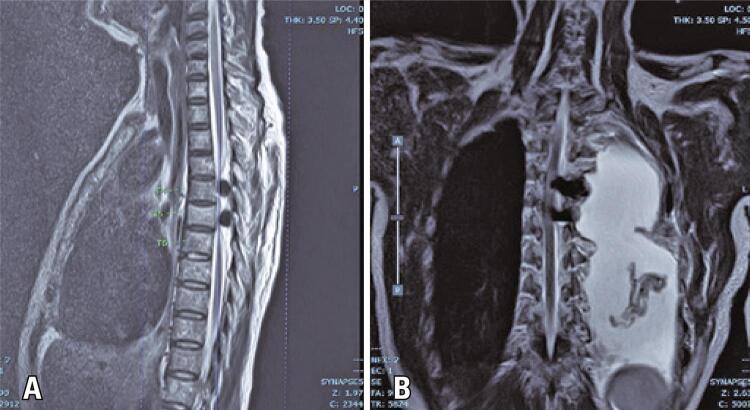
Sagittal (A) and coronal (B) T2 magnetic resonance imaging showing T4 and T5 hypointense mass
